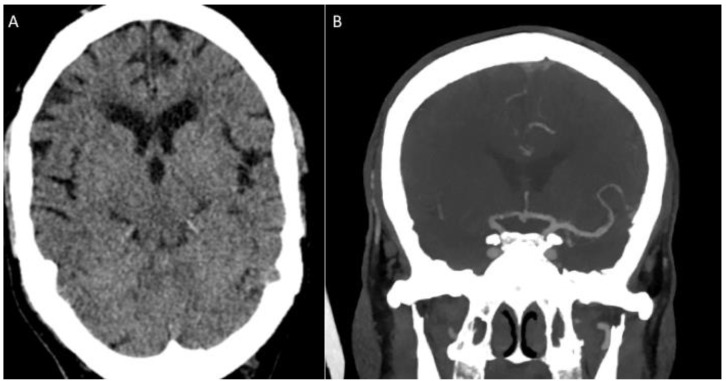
A male patient with 2 h history of acute left-sided weakness and an NIHSS score of 17. Non-contrast CT scan (A) revealed some subtle loss of grey–white matter differentiation involving the lentiform nucleus (ASPECT score 9), and CT angiography confirmed a right terminal ICA and M1 occlusion (B).
